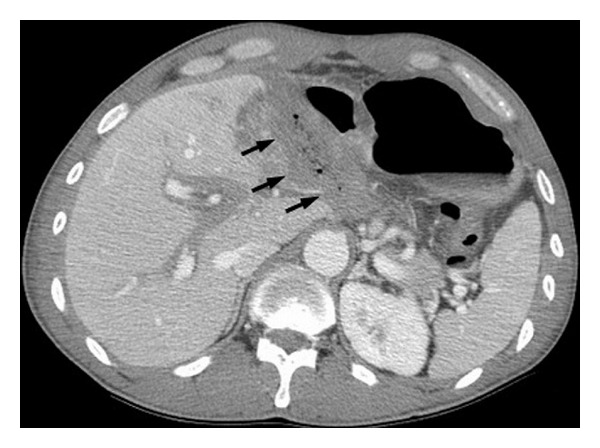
Abdominal computed tomography scan obtained after administration of contrast material shows mild biliary tree dilatation and a fluid collection with some air bubbles within.
